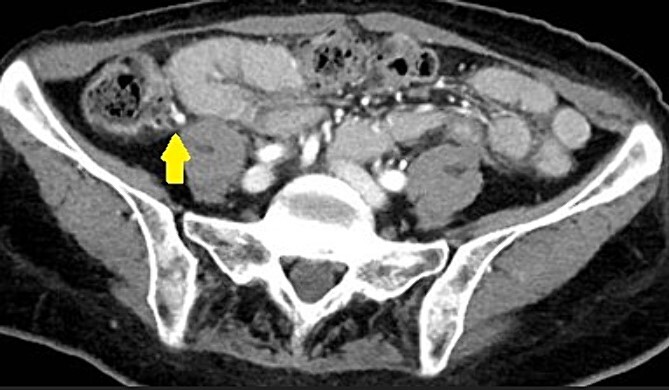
CT scan demonstrating an appendicolith (proven by pathology to be fecalith) at the base of the appendix.
